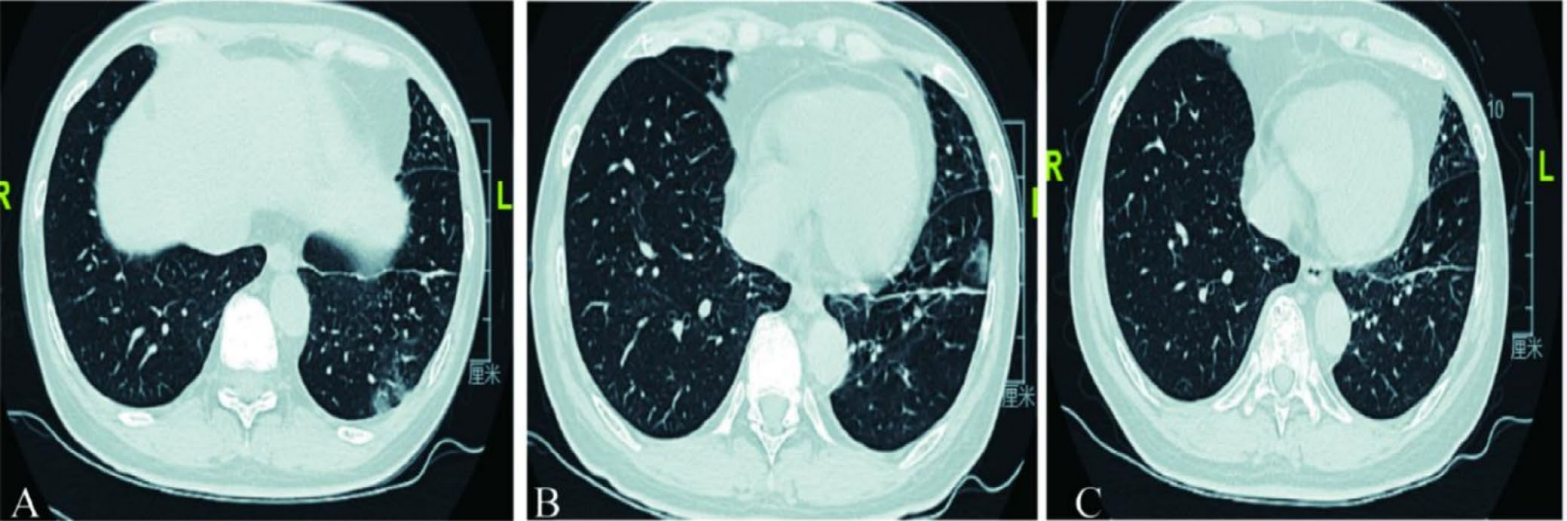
(A, B) HRCT (2nd degree ILD). (C) HRCT (ILD remission after methylprednisolone treatment).
